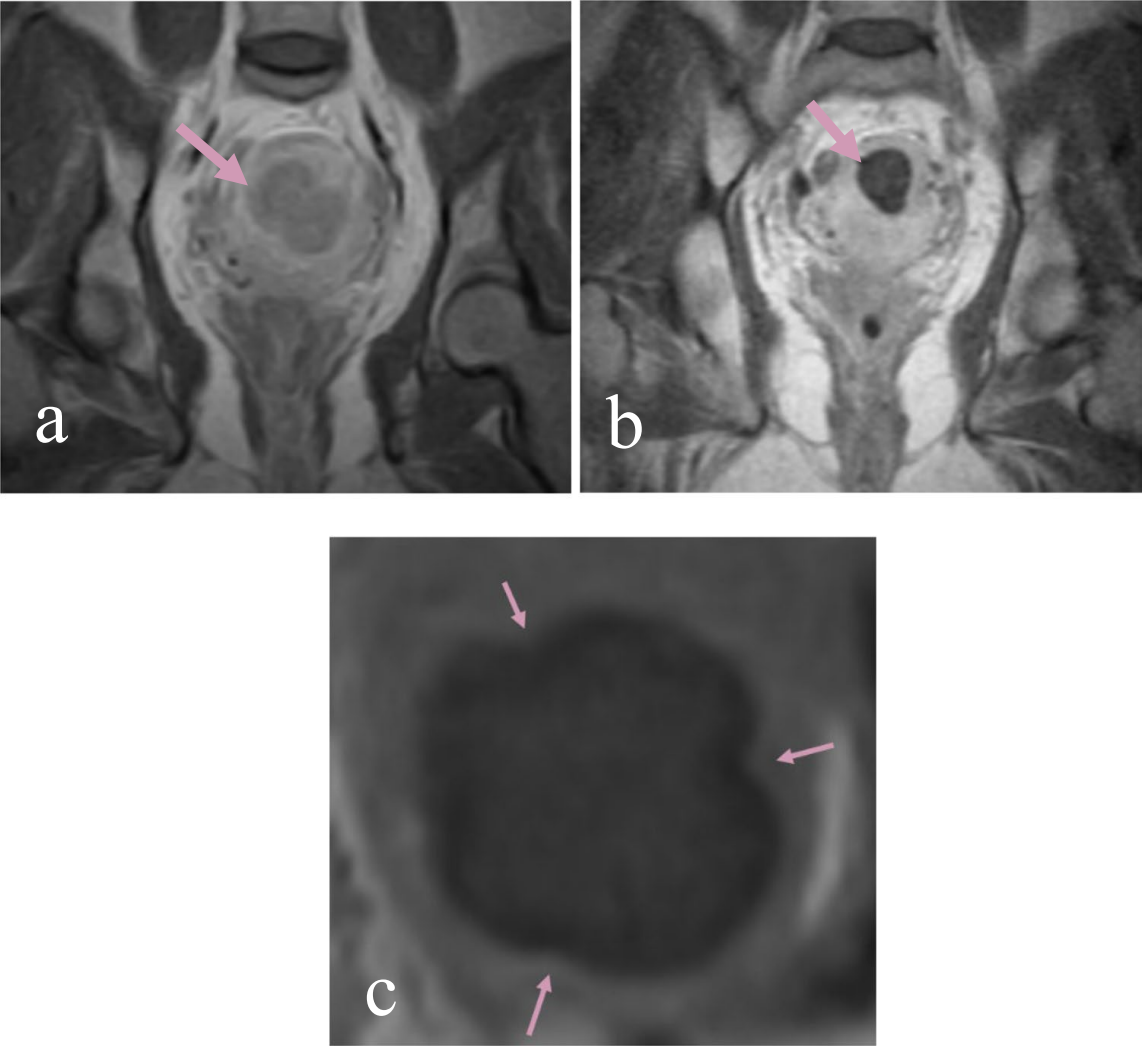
MRI—gadolinium-enhanced fat-suppressed T1-weighted images of a uterine fibroid a before the treatment—arrows point to the treated fibroid; b 12 months after the treatment; c 1 day after the treatment—arrows mark the sites at which sutures were placed with the PIFI system
